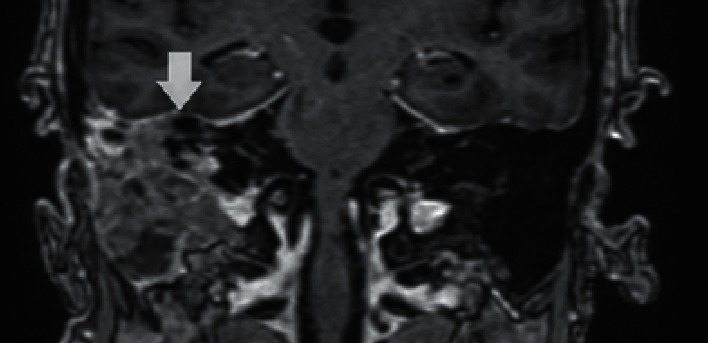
Initial coronal MRI demonstrating a heterogenous tissue mass in the right middle ear mastoid up to the dura of the middle cranial dura (white arrow).
